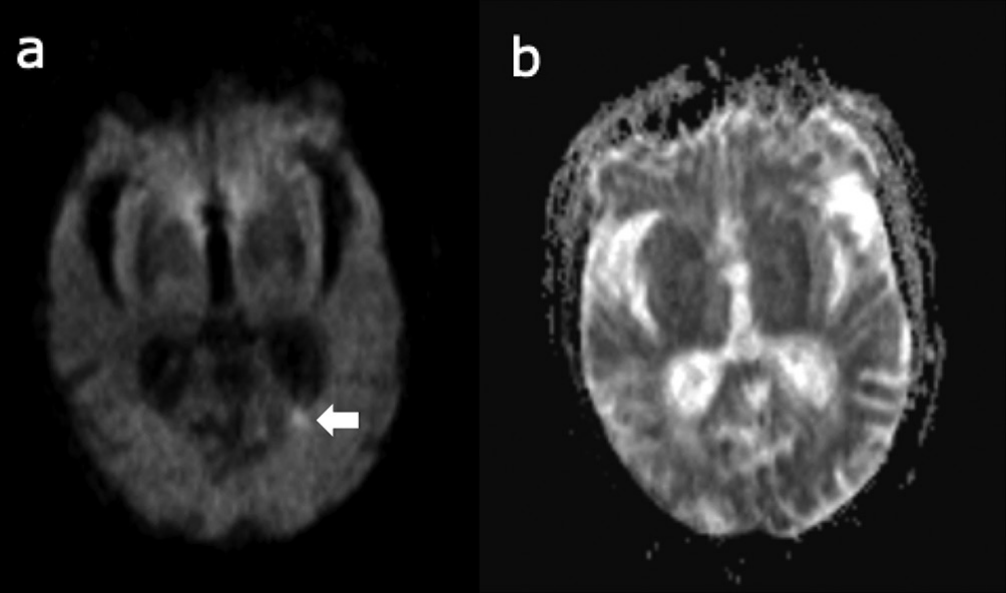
(a) DWI showing a small left occipital lesion (arrow); (b) ADC map does not depict a clear lesion corresponding to that seen on DWI. ADC, apparent diffusion coefficient; DWI, diffusion-weightedimaging.
